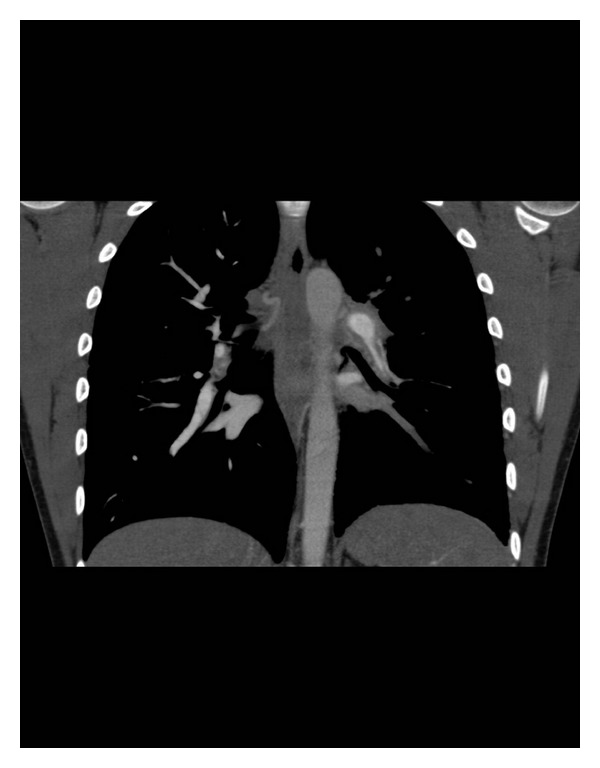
Coronal CT Image Demonstrating Pulmonary Arterial Thromboembolic Disease.
